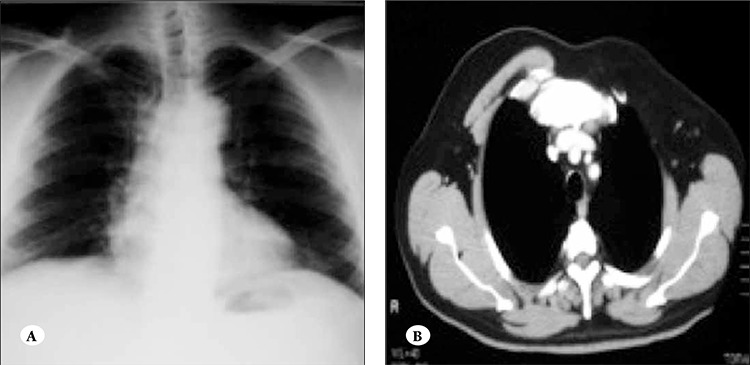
(A) Chest X-ray shows hyperlucency on the left side of the chest. (B) CT image shows agenesis of the left pectoralis major muscle.
